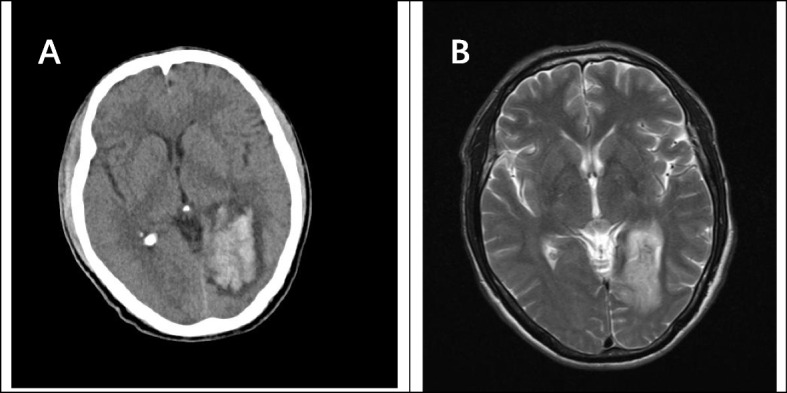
Imaging of hemorrhage in brain abscess. A Left occipital lobe cerebral hemorrhage focused on CT. B Corresponding brain abscess site before upper cerebral hemorrhage on MRI
